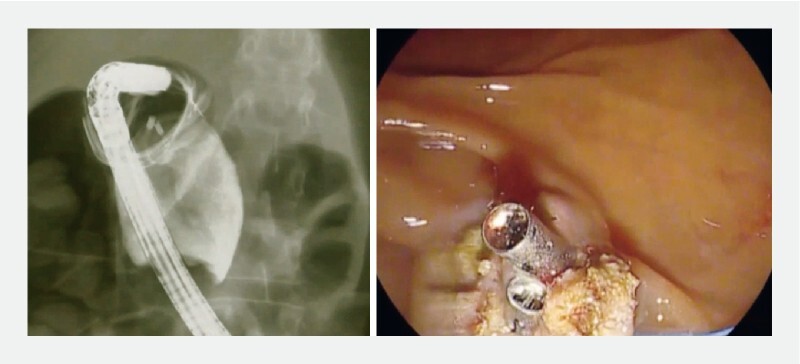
At the end of resection, through-the-scope endoclips were placed. No parietal perforation on contrast X-ray evaluation was observed.
